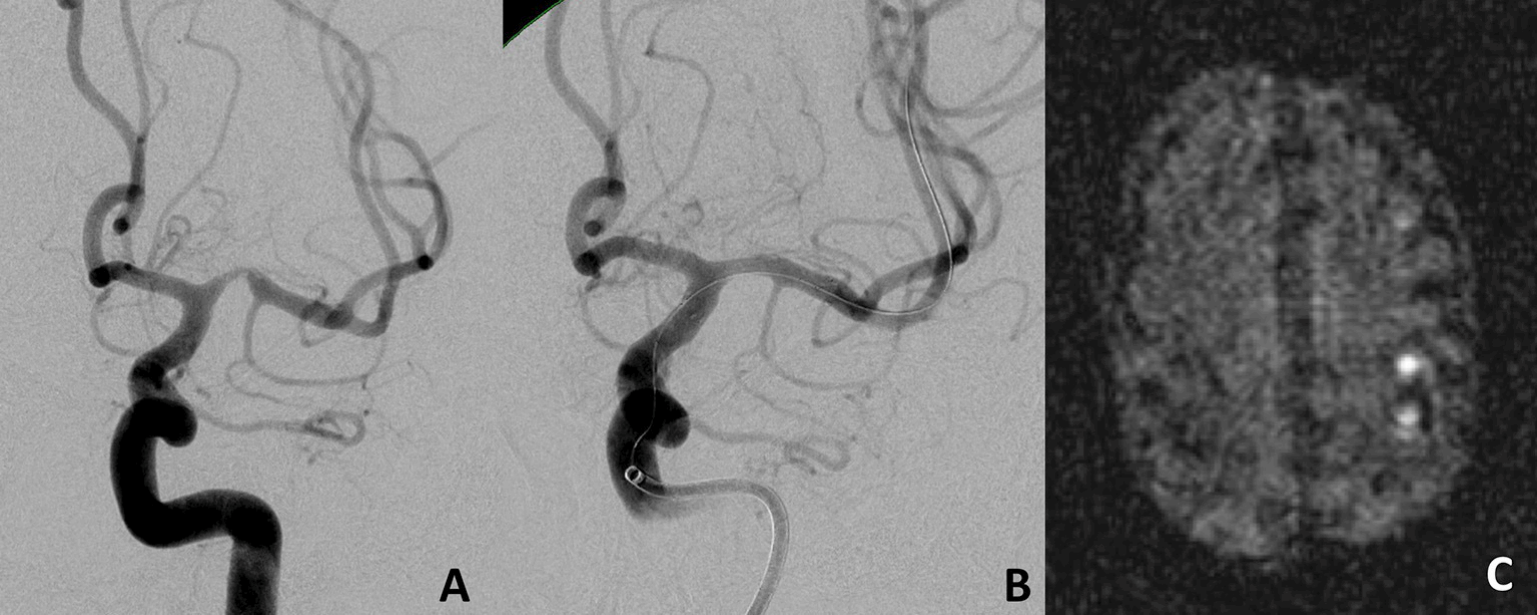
Proximal left middle cerebral artery M1 stenosis before (A) and after (B) Stent-PTA treatment. MRI at day 1 after the procedure showed asymptomatic cortical embolic infarctions in the left middle cerebral artery territory (C).
